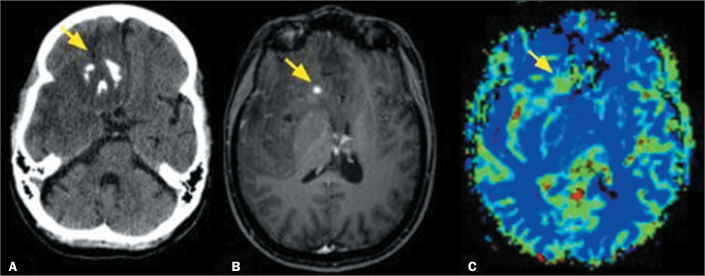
A 26-year-old female patient. A computed tomography scan (A) and MRI
